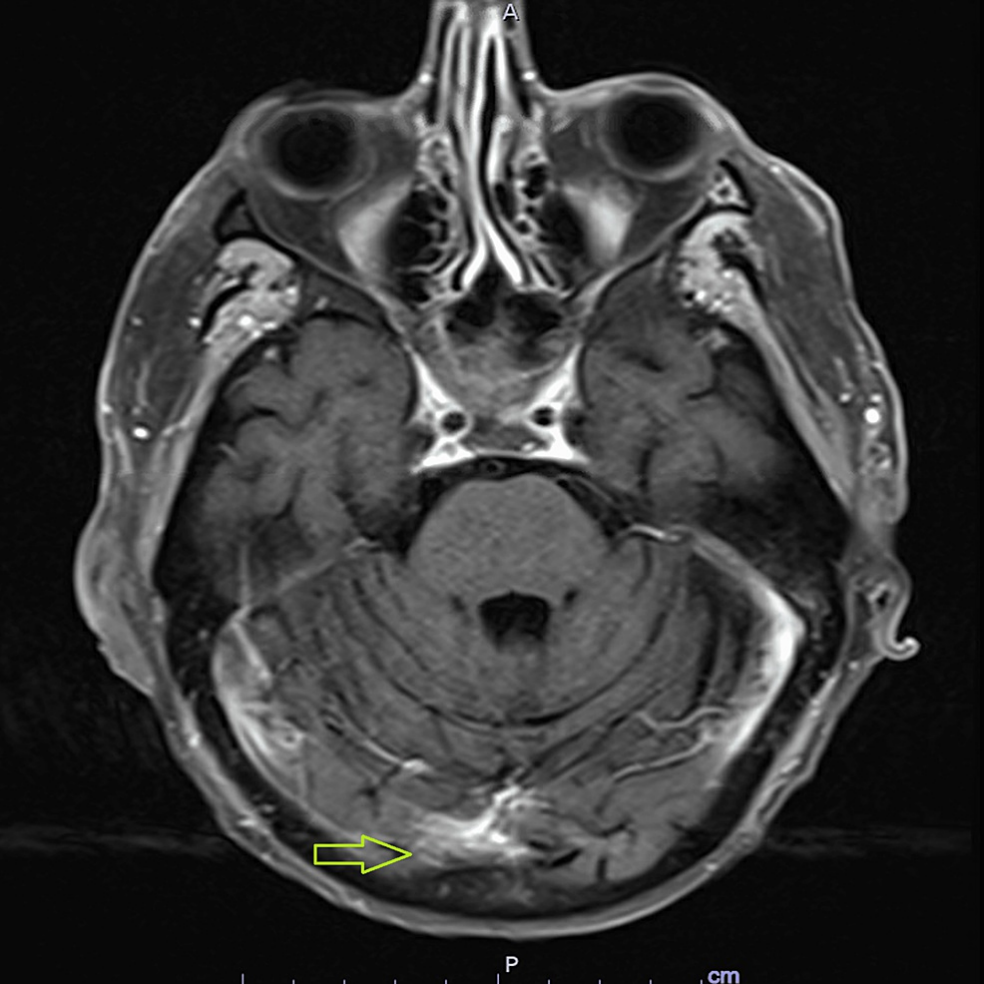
MRI of the brain demonstrating leptomeningeal enhancement of the superior and inferior cerebellar vermis
